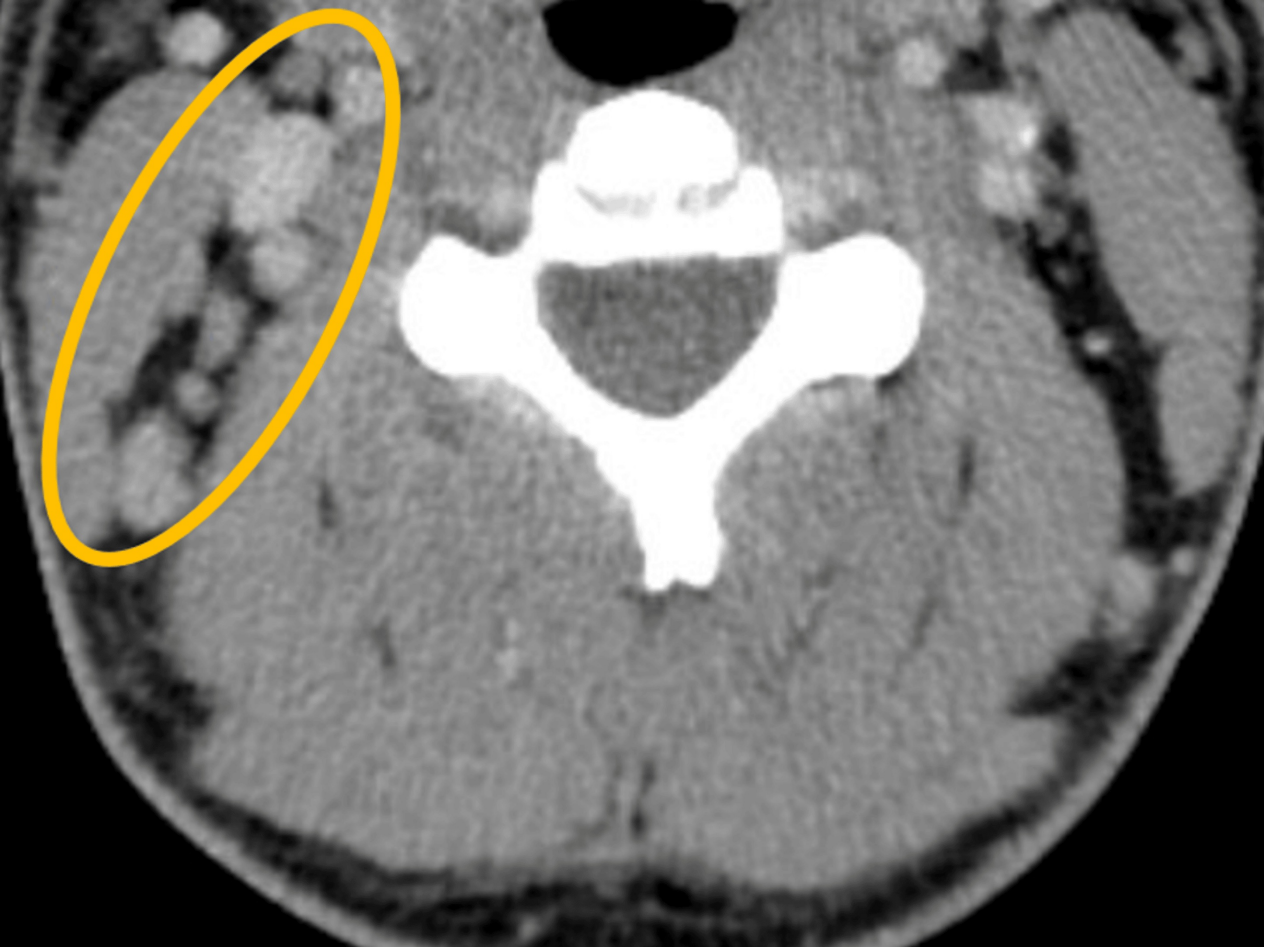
Axial image from CT soft tissue neck with intravenous contrast showing right-sided retro-auricular lymphadenopathy.
